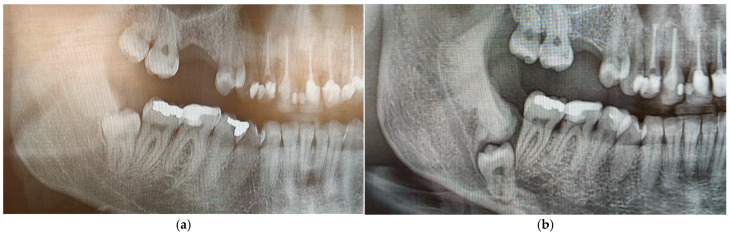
Radiographic comparison of the condition from three years ago (April 2022) (a) and the current state (June 2025) (b), showing a difference in the depth of impaction of tooth 48 and the size of the bony lesion.
